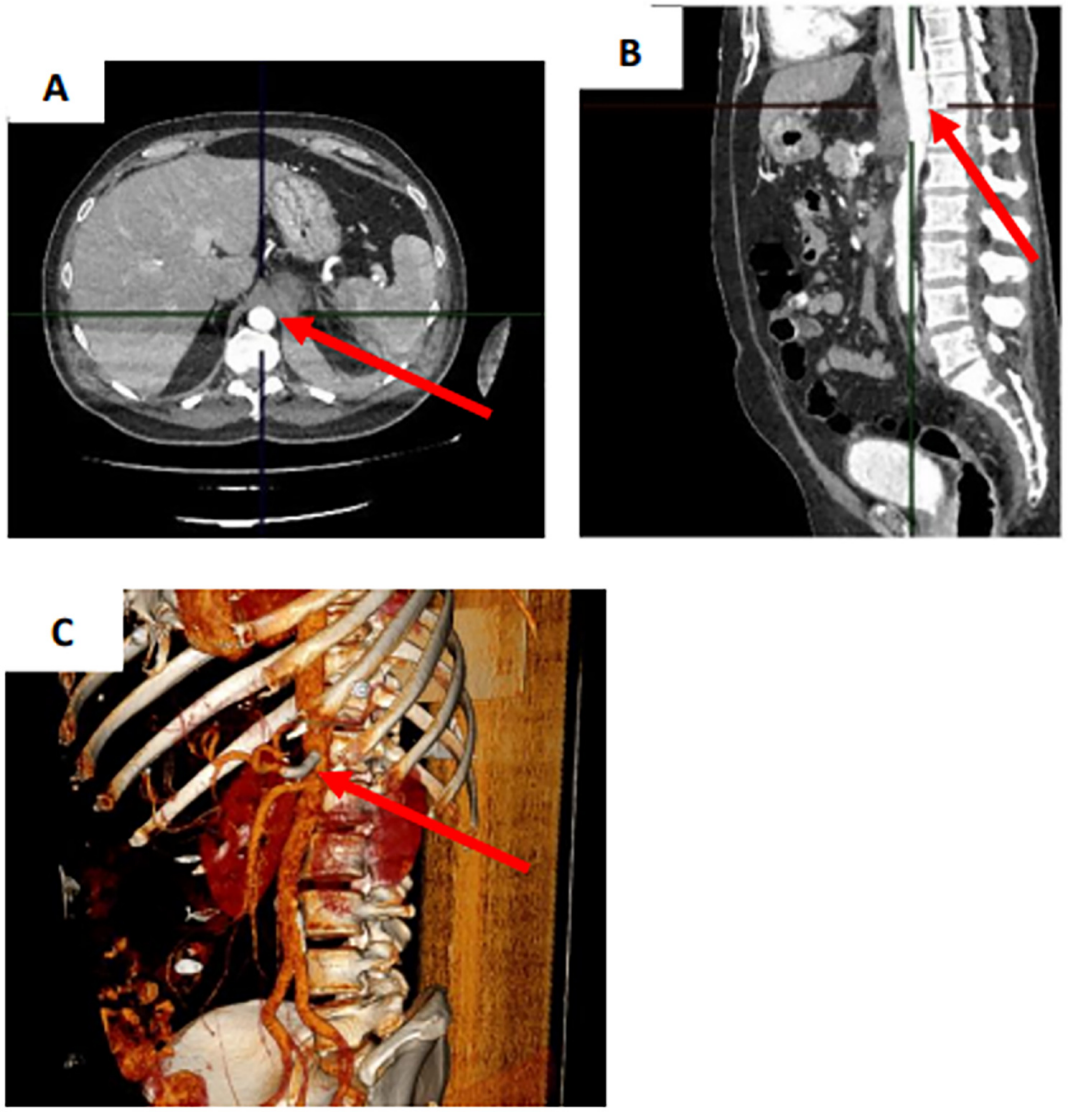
Control CT scan of the abdomen with intravenous contrast on the next day after stent-graft implantation. (A) Transverse projection. (B) Sagittal projection. (C) 3D model. CAA (arrow).
